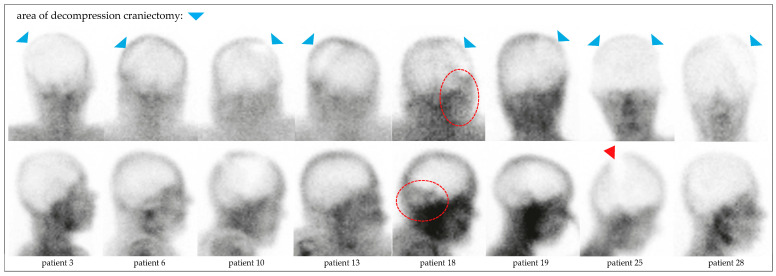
Planar scintigraphic images of all patients who underwent DC, posterior (upper row) and right lateral (lower row) views. The craniectomy defects (blue arrowheads) were best visualized from the posterior view. In patient no. 18, activity was seen projecting onto the cerebellum (red circle), which was further evaluated by SPECT/CT (see also Figure 4). In patient no. 25, the stripe of low uptake on the lateral view (red arrowhead) correlated with the long postoperative scalp suture following bilateral DC.
